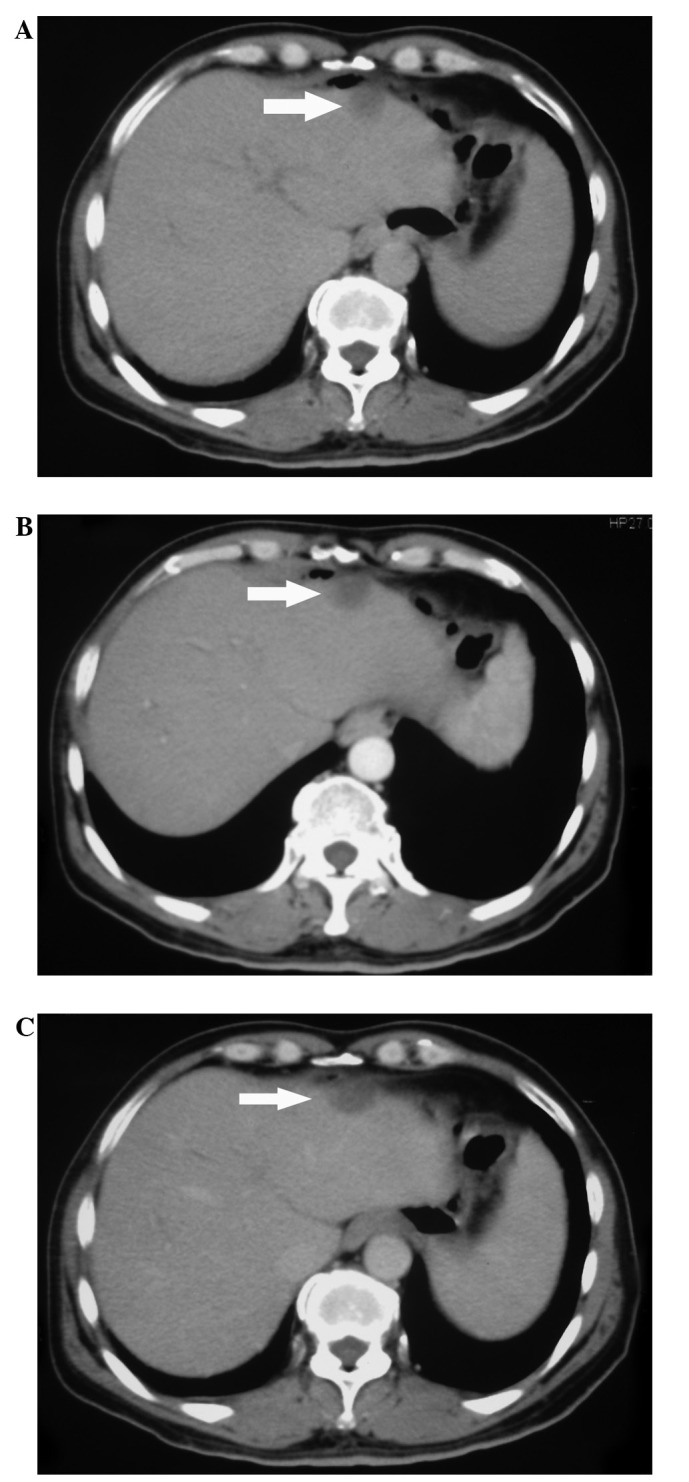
(A) Computed tomography (CT) scan showing the low-density liver lesion on the surface of the left lateral lobe and the adhesion to the intestine in the local area. (B and C) Contrast-enhanced CT scan demonstrating no marked change in intensification in the arterial and venous phases.
